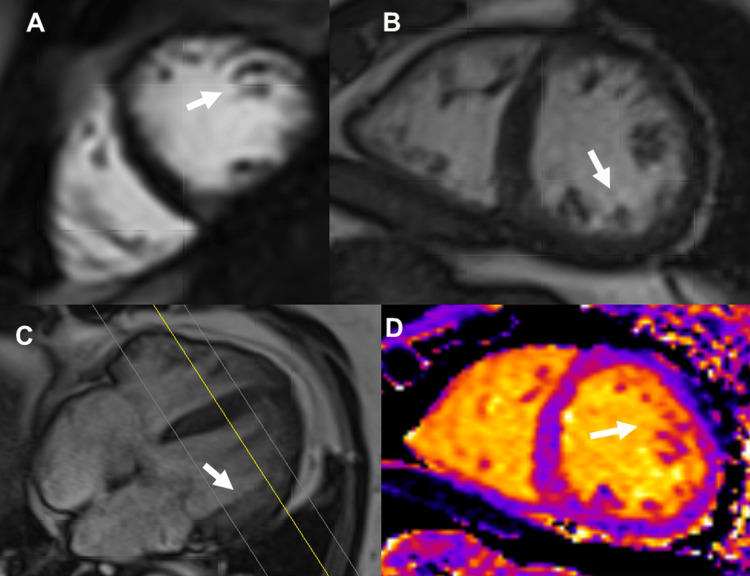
Cardiac MRI images Cardiac MRI showing extensive hypertrabeculation of the left ventricular myocardium distally to the papillary muscles. Panel A: Short-axis view (DYN_ sBTFE_5sl); Panel B: Short-axis view (Trufi Cine SAX); Panel C: Long-axis view (Trufi Cine 4CH); Panel D: Axial view (T1 Map SAX MOCO T1). After the application of the contrast medium, no LGE could be documented.
